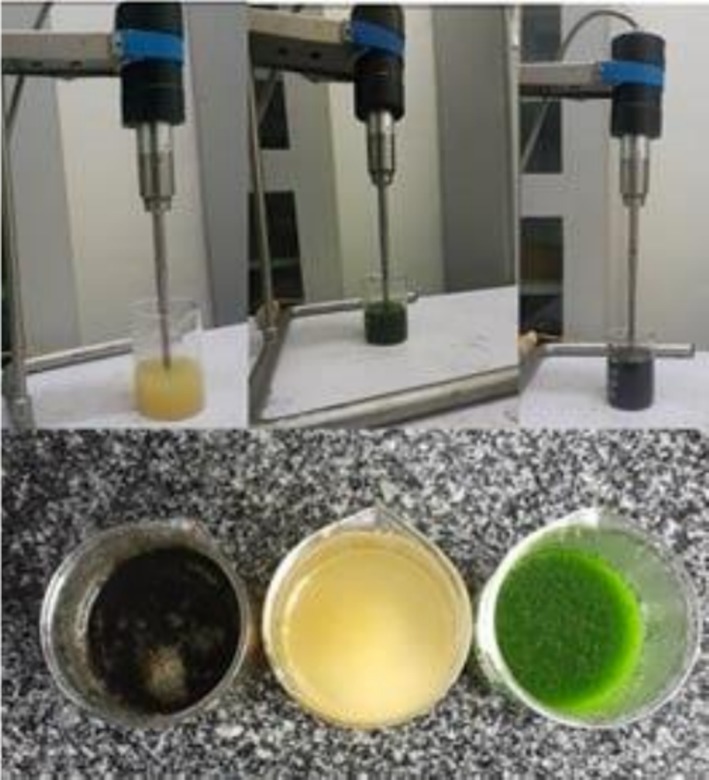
Ultrasonic extraction of the selected plants.
